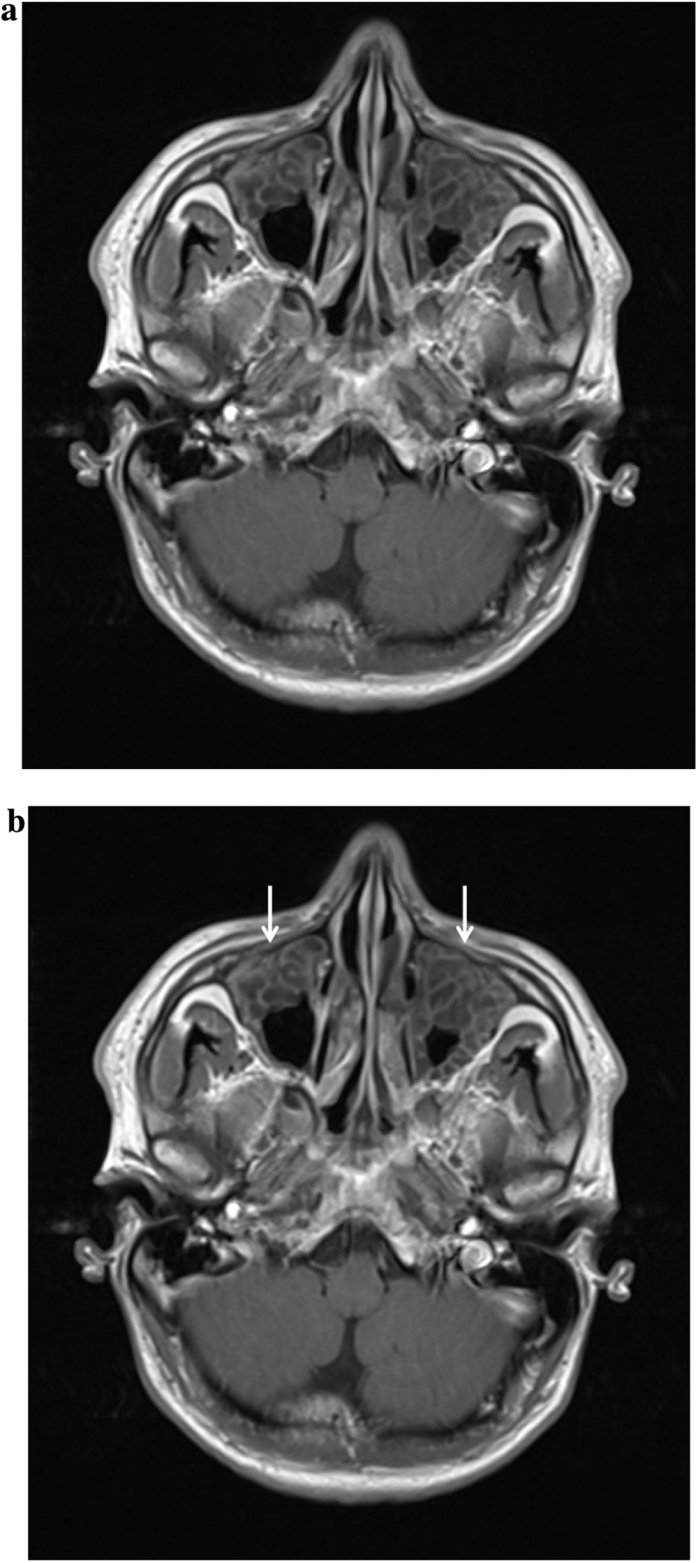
(a) MRI Head (T1 weighted with contrast) showing extensive loculated sinusitis (unmarked). (b) MRI Head (T1 weighted with contrast) showing extensive loculated sinusitis (marked).
